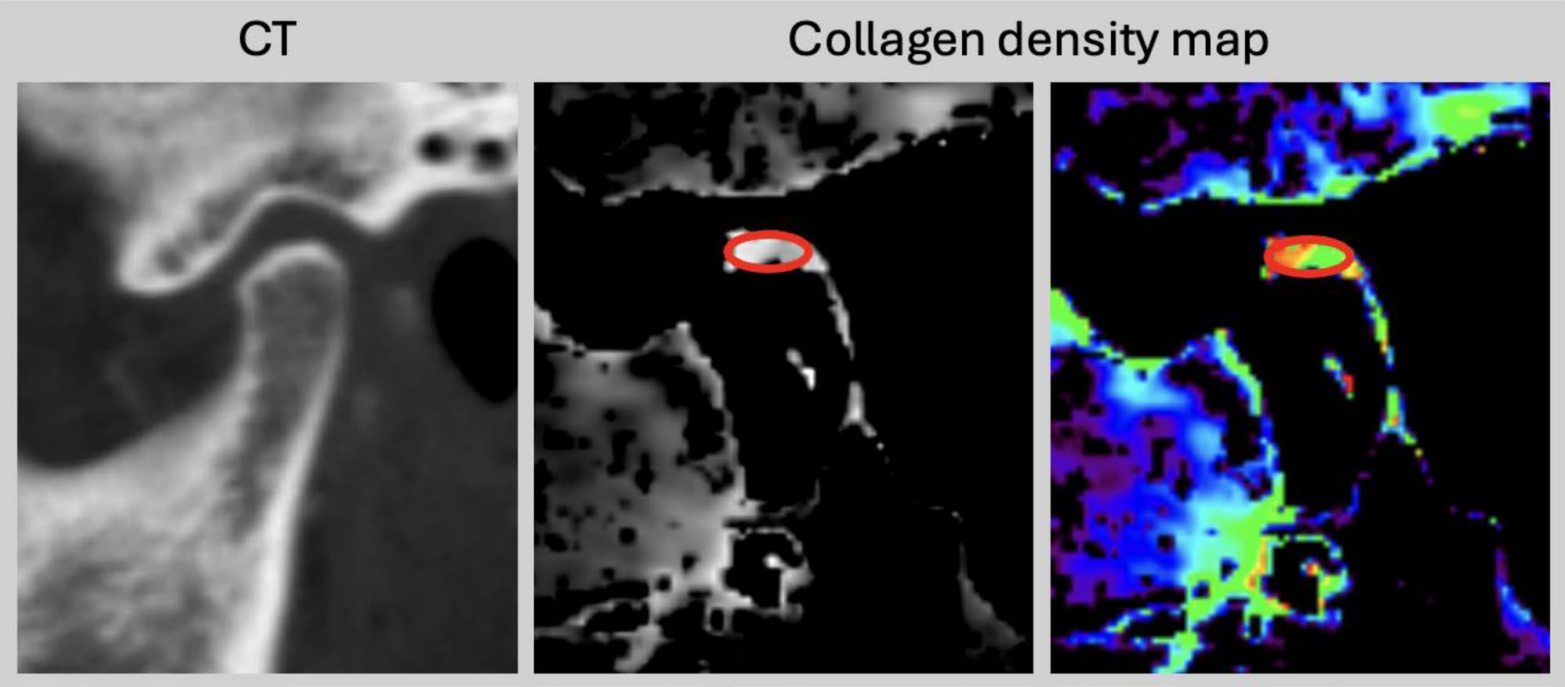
Quantitative measurement. Example image of clinical CT and collagen map with region of interest placement (red oval) in the temporomandibular joint
